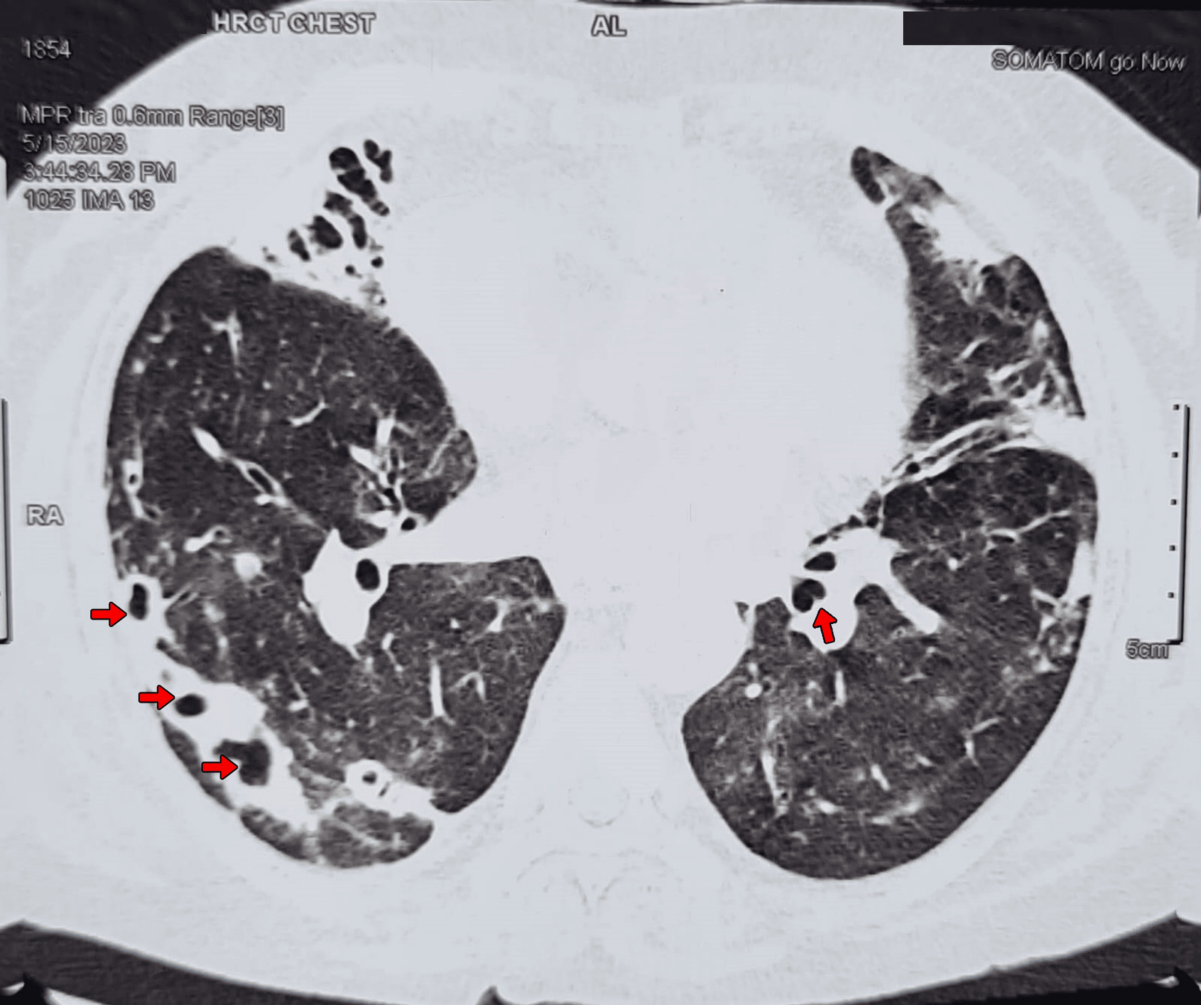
HRCT chest (axial view) – bilateral pulmonary cavities High-resolution computed tomography (HRCT) chest (axial plane) demonstrating multiple thick-walled cavitary lesions in both lungs (red arrows). These findings raised the differential diagnosis of granulomatosis with polyangiitis versus secondary infection in the context of ANCA-negative vasculitis. ANCA: anti-neutrophil cytoplasmic antibody
